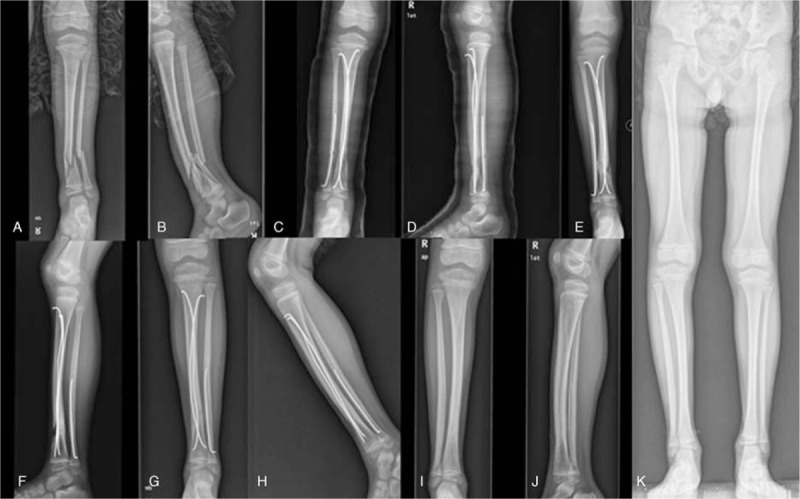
A 7.4-year-old boy suffered traffic accident. A, B, Anteroposterior and lateral views of the severe right oblique DTDMJ fracture, multifragmented open fracture, Gustilo type I. C, D, Tibia was fixed with 2 nails, fibula with 1 nail. E, F, 6 months after surgery, the fracture with radiographic evidence of delay union. G, H, 10 months after surgery, the fracture showed evidence of healing. I, J, 16 months after surgery, no growth arrest or disturbances by radiological assessment. K, 24 months after surgery, standing weight-bearing view showed 10 mm overgrowth on the right leg.
